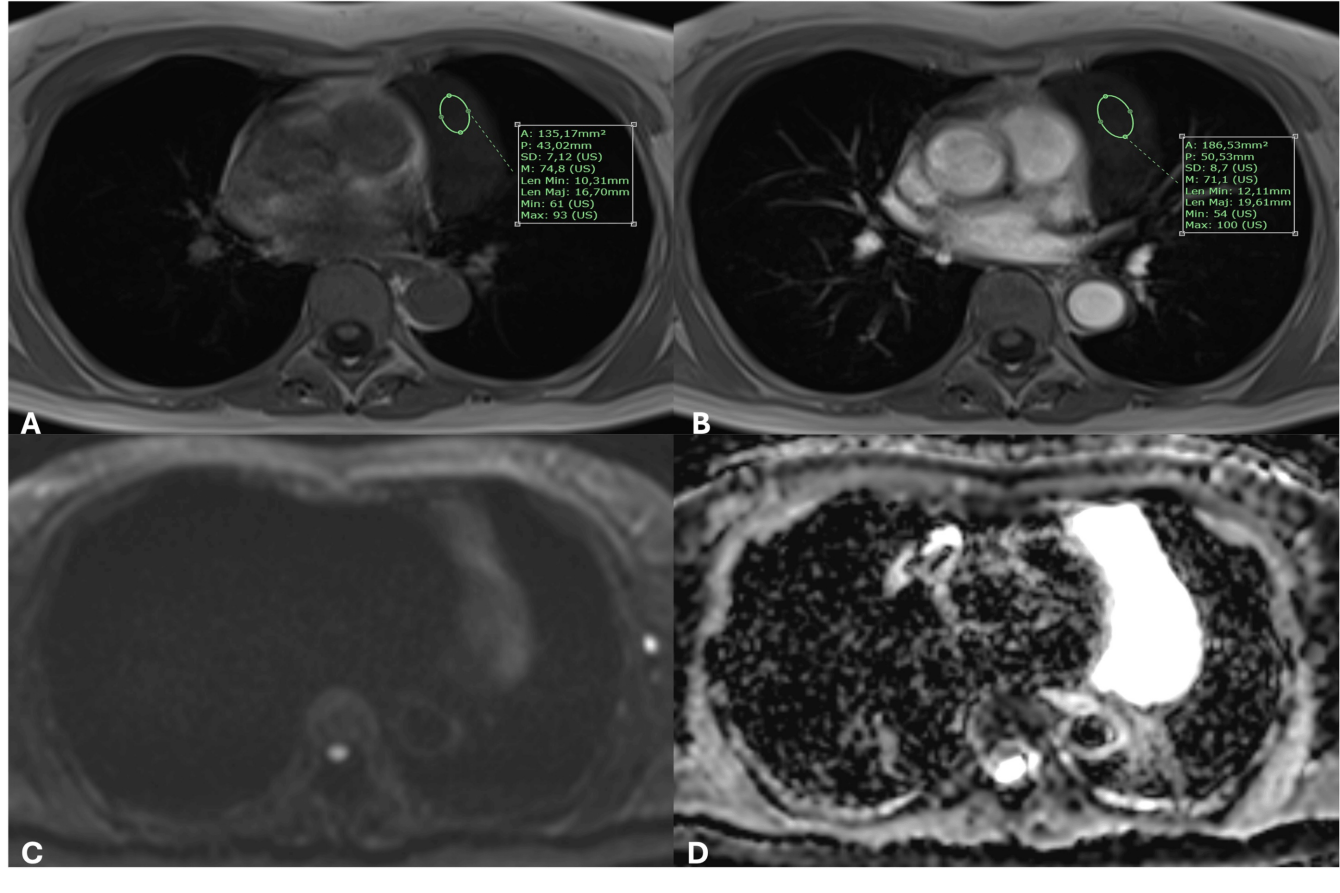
(A) T1-weighted sequences with intravenous contrast administration (gadolinium) do not reveal any enhancement. (B-D) DWI and ADC map show no signs of restriction to diffusion within the lesion. Overall, no mass effect, invasion of adjacent structures, or pericardiac effusion is noted. DWI: diffusion-weighted imaging, ADC: apparent diffusion coefficient
